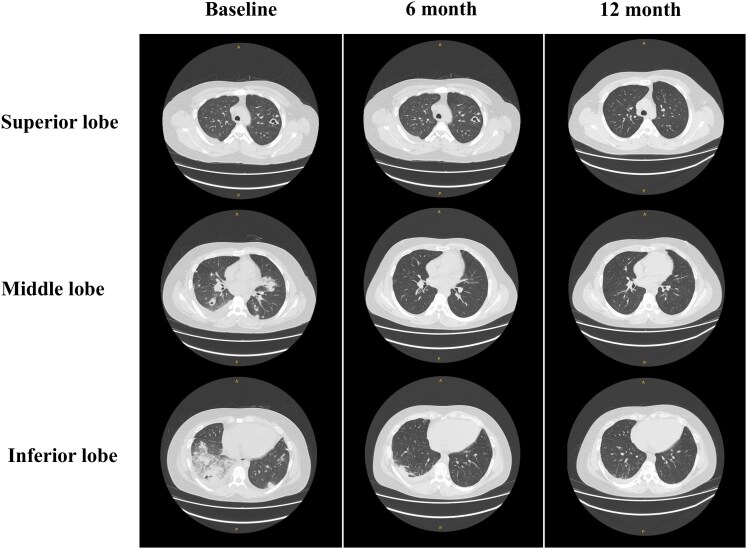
Chest computed tomography at baseline demonstrated multifocal pneumonia and lung abscesses, consistent with septic pulmonary embolic disease. Serial follow-up computed tomography showed improvement in lung involvement after antibiotic therapy for infective endocarditis.
